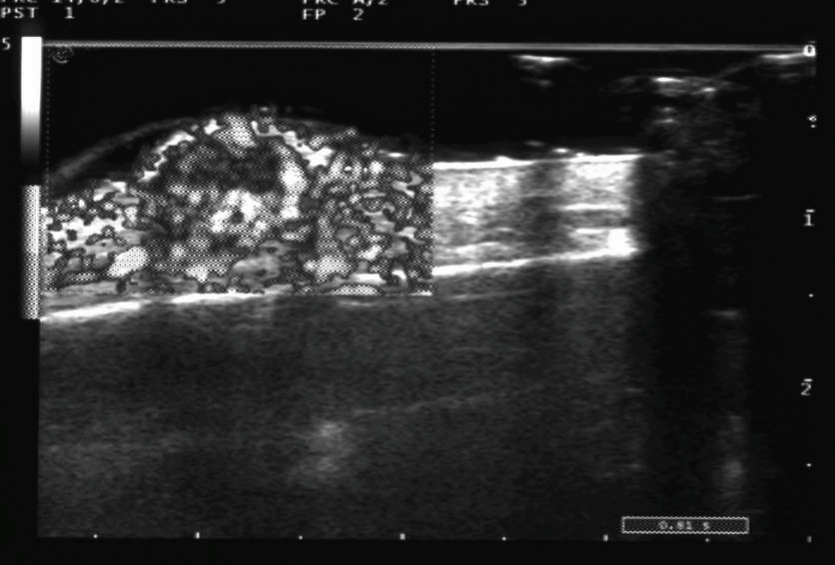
Vascular aspects of AIDS-KS. AIDS-KS lesion, with evident vascularisation; the monochromatic color power Doppler indicates marked vascularisation of the periphery of the nodule, with a ring-like pattern and a hypovascular central area.
